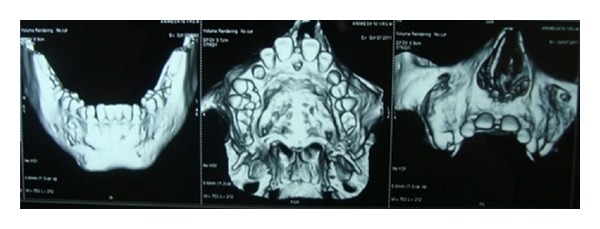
CT scan showing 3D reconstruction (Case 1).
